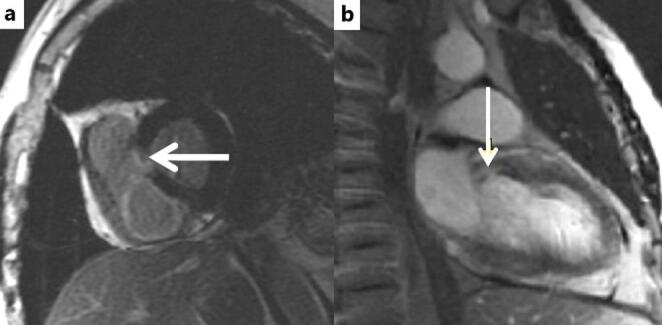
Diabetic cardiomyopathy. a–b MRI (late gadolinium enhancement; LGE). A man in his 50s with type 2 DM, whose left ventricular ejection fraction decreased to approximately 50%. Cardiac MRI a–b reveals delayed enhancement in the anterior septum at the base of the heart (arrow). The native T1 value was 1221 ms, which was within the normal range. CT Angiography shows no significant stenosis in the coronary arteries (data not shown), indicating diabetic cardiomyopathy independent of the coronary artery distribution. Abbreviations: LGE, late gadolinium enhancement; CT, computed tomography; DM, diabetes mellitus; MRI, magnetic resonance imaging
